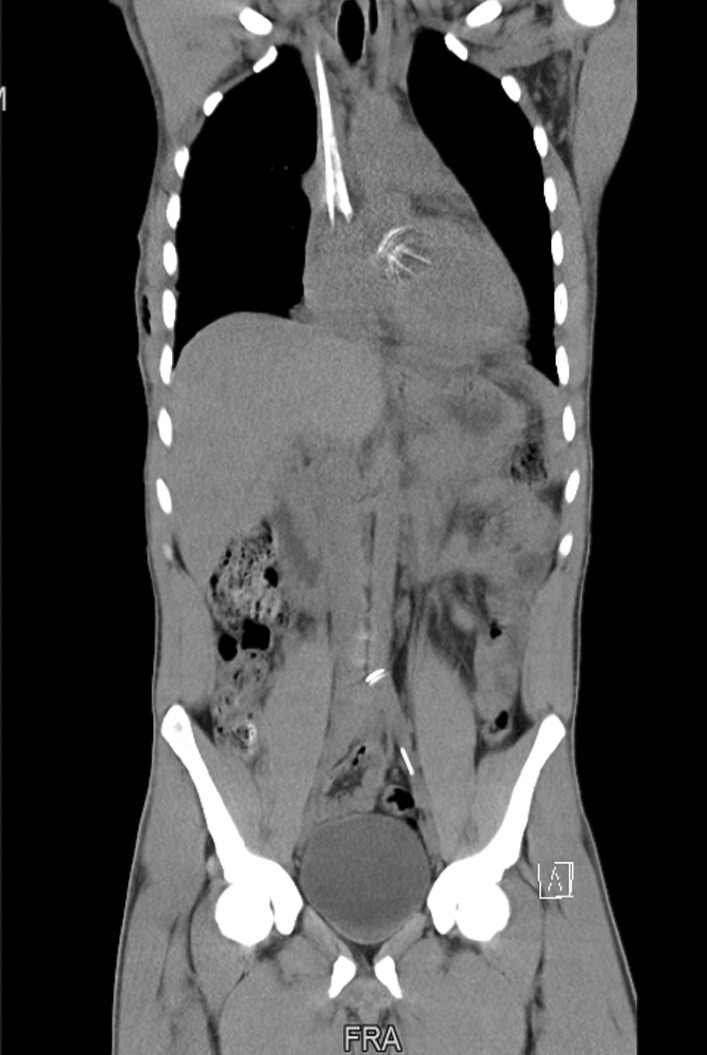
Computed tomography of the whole body showing the mechanical mitral valve leaflet at the abdominal aortic bifurcation and left external iliac artery
